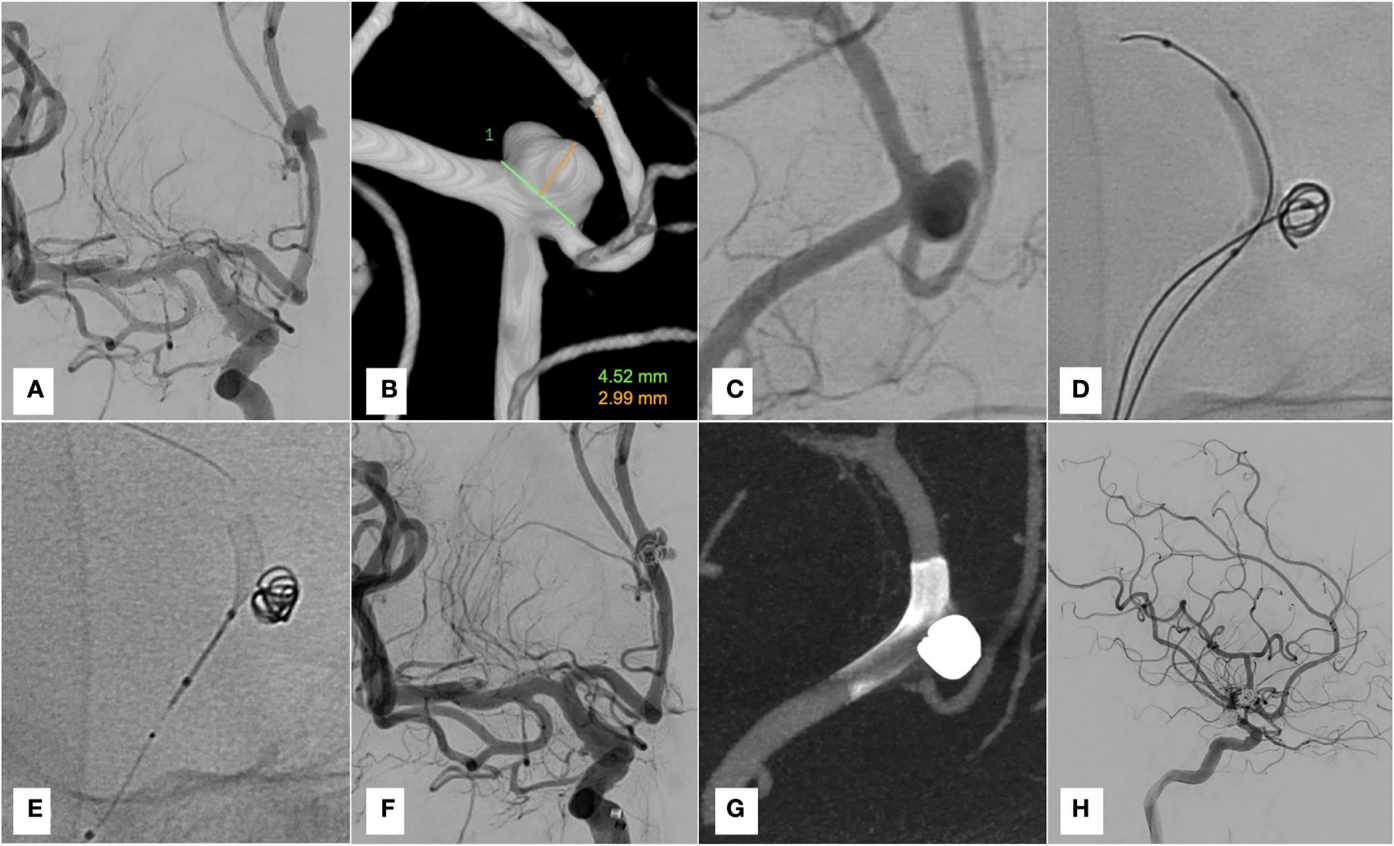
Case 1. (A–C) Pre-treatment DSA and 3D reconstruction. (D) Coiling with Scepter C inflated. (E) Deployment of Derivo 2 through the dual lumen balloon. (F,G) Final run and Vaso-CT. (H) Six-month-DSA follow-up.
